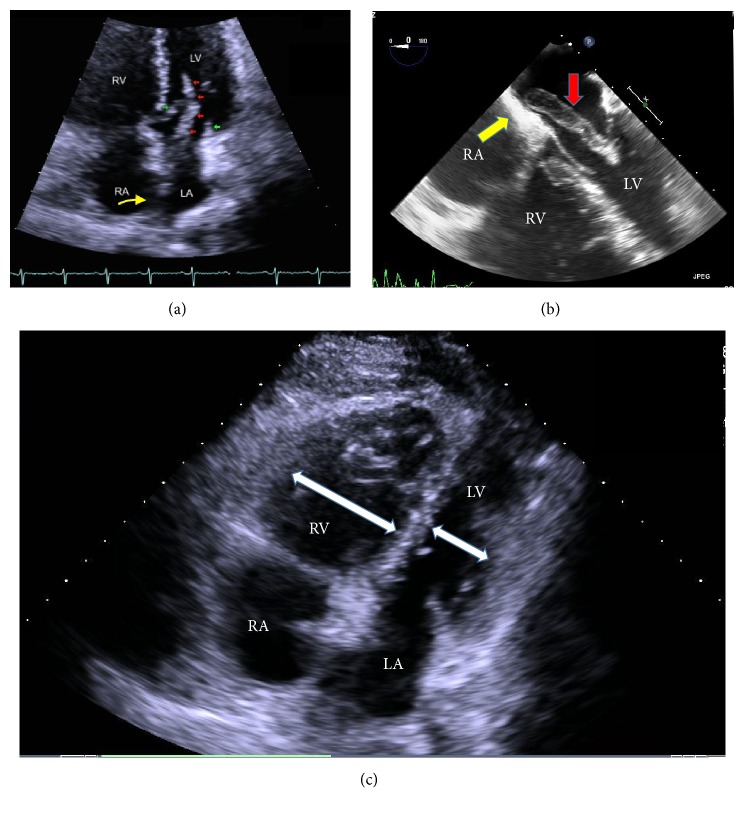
Echocardiography: (a) TTE apical four-chamber view demonstrating elongated LA thrombus (red arrows) prolapsing through PFO and LA into the LV during the diastole. Green arrows depict mitral valve leaflets. (b) The same clot is clearly visualized on TEE attached to interatrial septum. Yellow arrow depicts the location of PFO. (c) TTE subcostal view enlarged right heart chambers exceeding their left counterparts in size.
